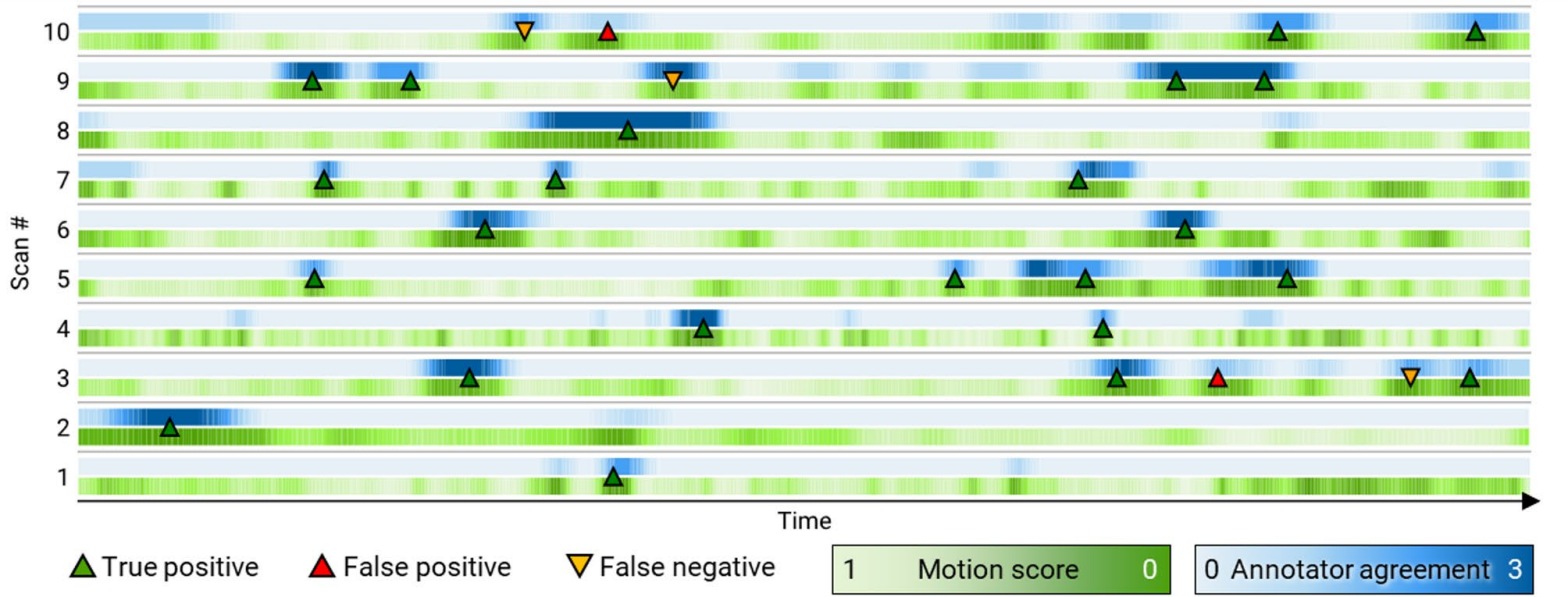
Stationary position detection evaluation on multispectral optoacoustic-ultrasound (MS-OPUS) scans. Three human annotators reviewed US videos of ten MS-OPUS scans (Dataset 1) and identified 25 stationary positions (blue). *Motion scores* of those scans were also computed (green) and the most optimal frames were selected (n=$$\:{N}_{peaks})$$, such that $$\:{N}_{peaks}$$ matches the number of true stationary positions identified by the human annotators. The selected frames are marked by green (true positive) and red triangles (false positive). Stationary positions missed by our algorithm (false negatives) are marked by yellow upside-down triangles.
